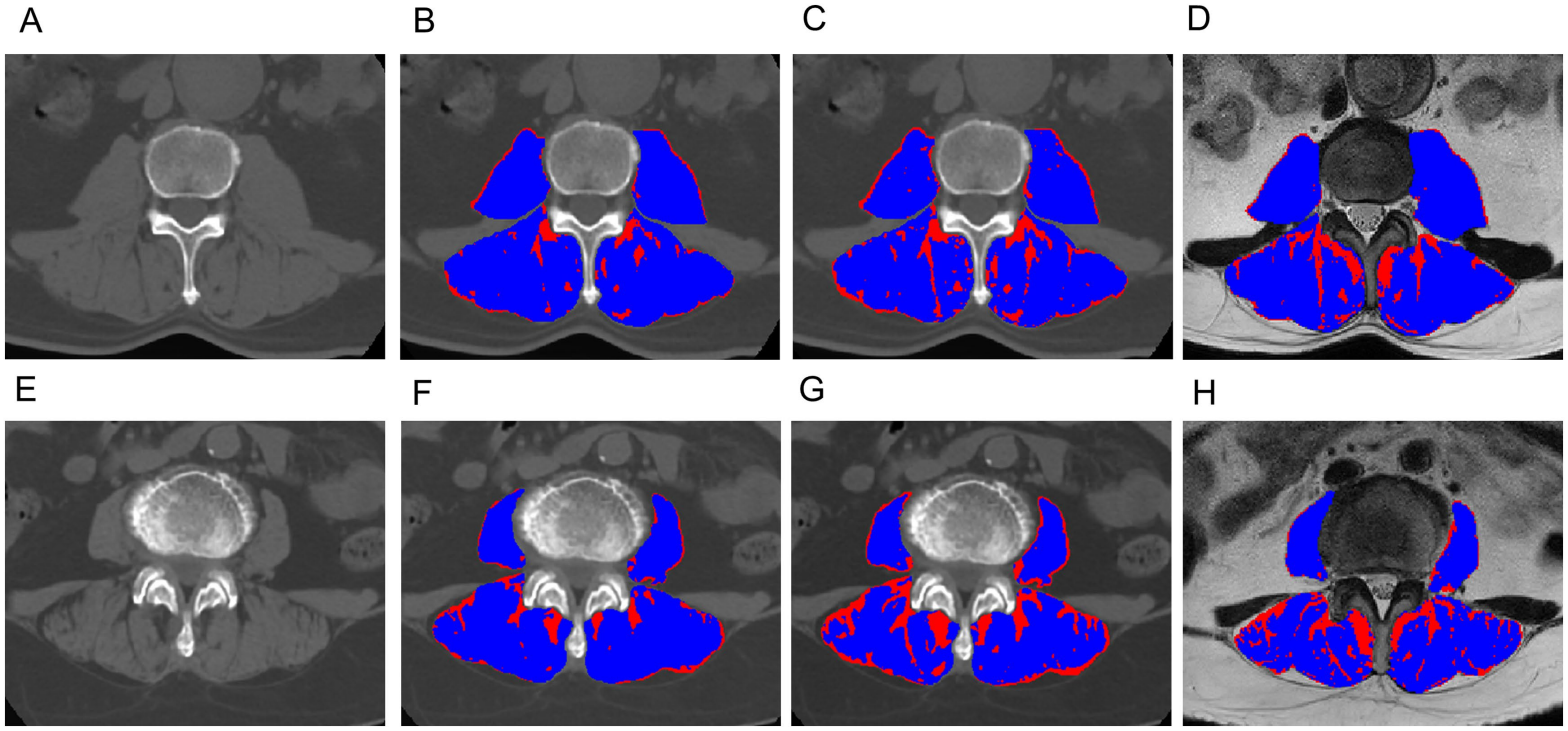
Exemplary slices showing the differentiation between muscle (blue) and fat (red) obtained with the CT-based Gaussian Mixture Model (B,F), CT-based Otsu thresholding method (C,G) and MRI-based Otsu thresholding method (D,H), (A–D): A 62-year-old male with sarcopenia; (E–H): an 66-year-old female without sarcopenia. The AUC of the CT-based Gaussian Mixture Model and the Otsu model for predicting sarcopenia was 0.98 and 0.93, respectively. The AUC of the Gaussian mixture model and the Otsu model for predicting sarcopenia was 0.990 and 0.986, respectively.
